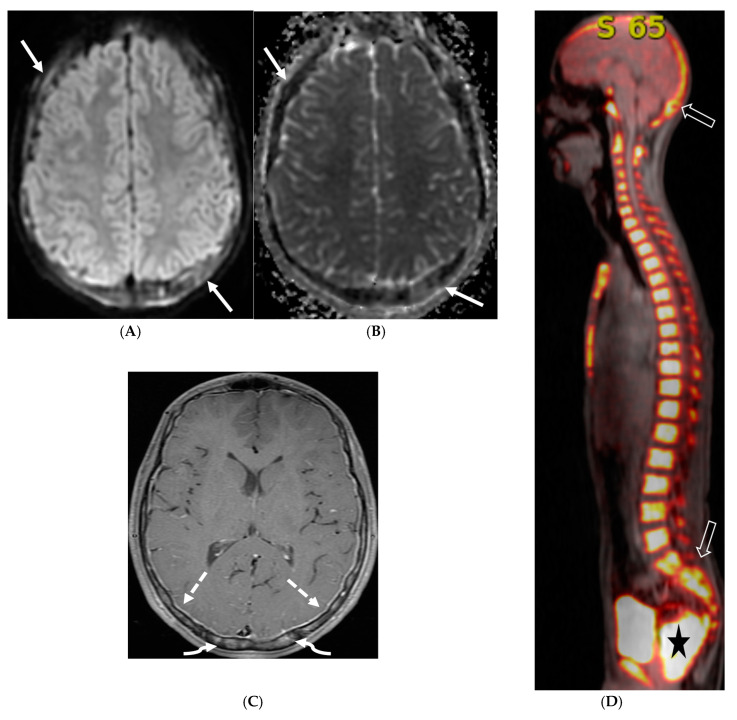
Axial DWI (A), axial ADC (B), axial T1 FS post contrast (C) and Fluorodeoxyglucose Positron Emission Tomography (FDG–PET) scan (D): 13-year-old male with bilateral leg pains, headache, fever and weight loss: Blood tests and CT scan were concerning for Burkitt’s lymphoma. There is heterogeneous calvarial bone marrow signal with restricted diffusion (arrows) and patchy enhancement (curved arrows). Diffuse thickening and enhancement of pachymeninges in the supratentorial compartment is noted (dashed arrows). Findings are most consistent with lymphomatous involvement. Multifocal diffuse/heterogeneous pattern of FDG uptake within the axial and appendicular skeleton and the calvarium (open arrows). Intense FDG avid uptake is seen in the presacral mass (star).
